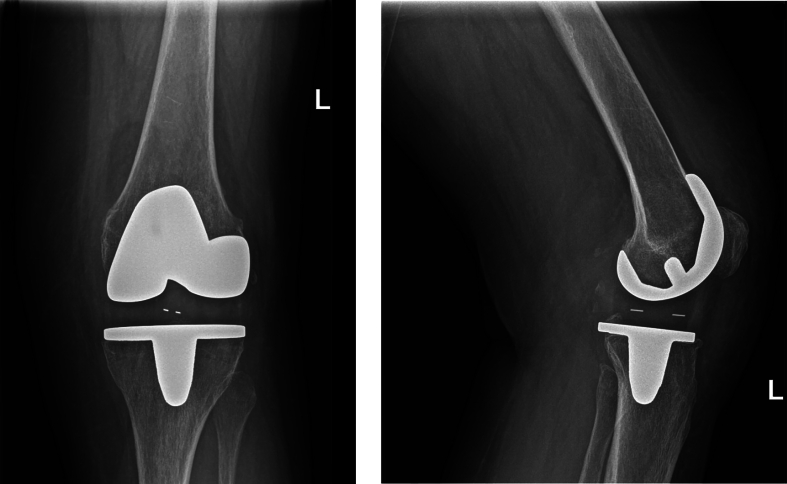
Case 2: conventional radiography images after revision surgery.
